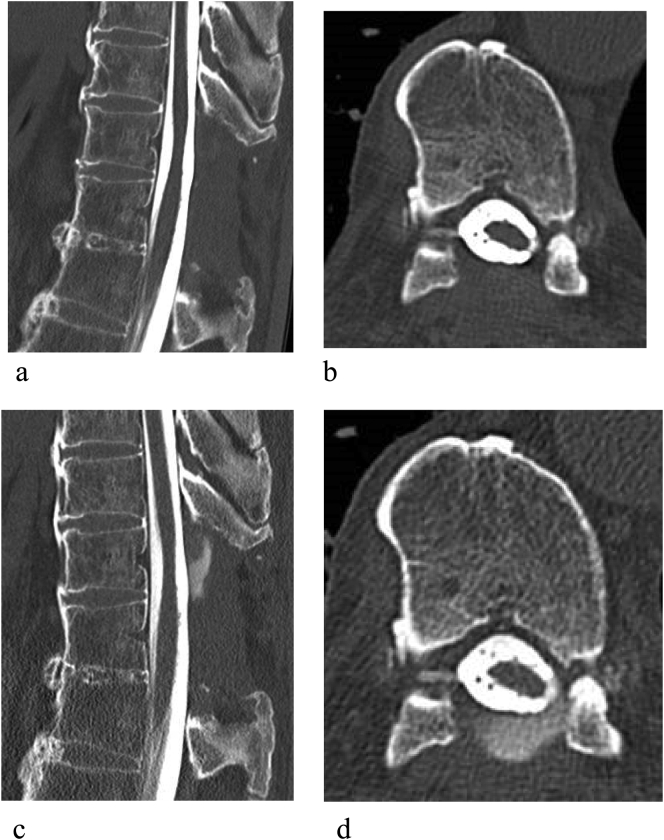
Computed tomography myelograms at T10–11. (a) Sagittal view. (b) Axial view. Delayed computed tomography myelograms show flow of contrast into the lesion at T10–11. (c) Sagittal view. (d) Axial view.
